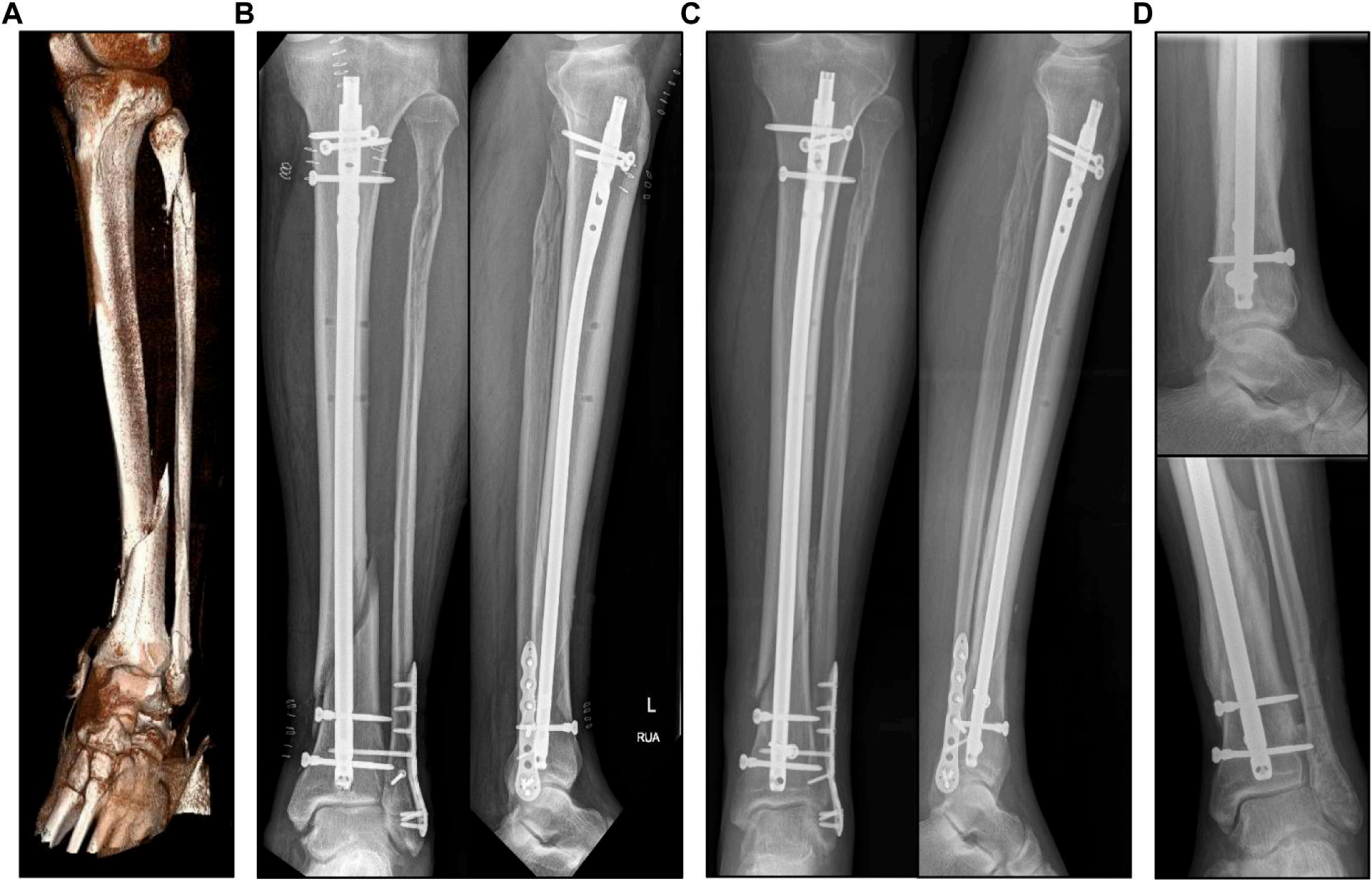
(A): 3D reconstruction of the injured lower leg showing diaphyseal distal tibia fracture and a proximal and distal fibula fracture. (B): X-rays antero-posterior and lateral view after surgical intervention by intramedullary nailing and plate osteosynthesis of the distal fibula fracture. (C): X-ray control 6 weeks after surgery. (D): Final X-ray approximately 7 months after surgery and shortly after plate removal at the distal fibula as well as removal of the set screw in the meantime. Note full osseous healing of the distal tibia and fibula fracture.
